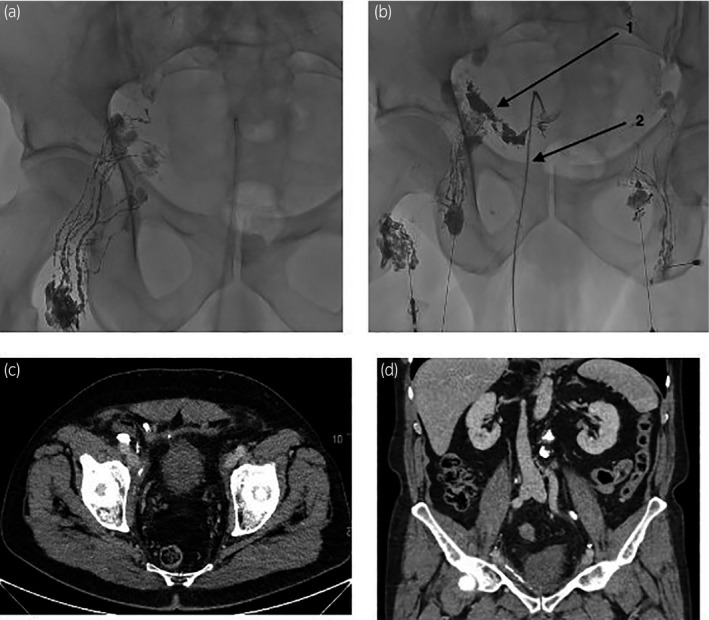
Patient 1: lymphangiography and follow‐up. (a) Right lymphangiography: no leak initially identified. (b) During left lymphangiography: leakage (1) on the right side showed on late images. The leak is directed toward the percutaneous catheter (2), and probably comes from external iliac lymph ducts. Puncture and glue/Lipiodol injection into the closest lymph node from the leak. (c, d) CT scan 10 weeks after the right lymphatic embolization (a, axial slice; b, coronal reformation). No residual lymphocele.
